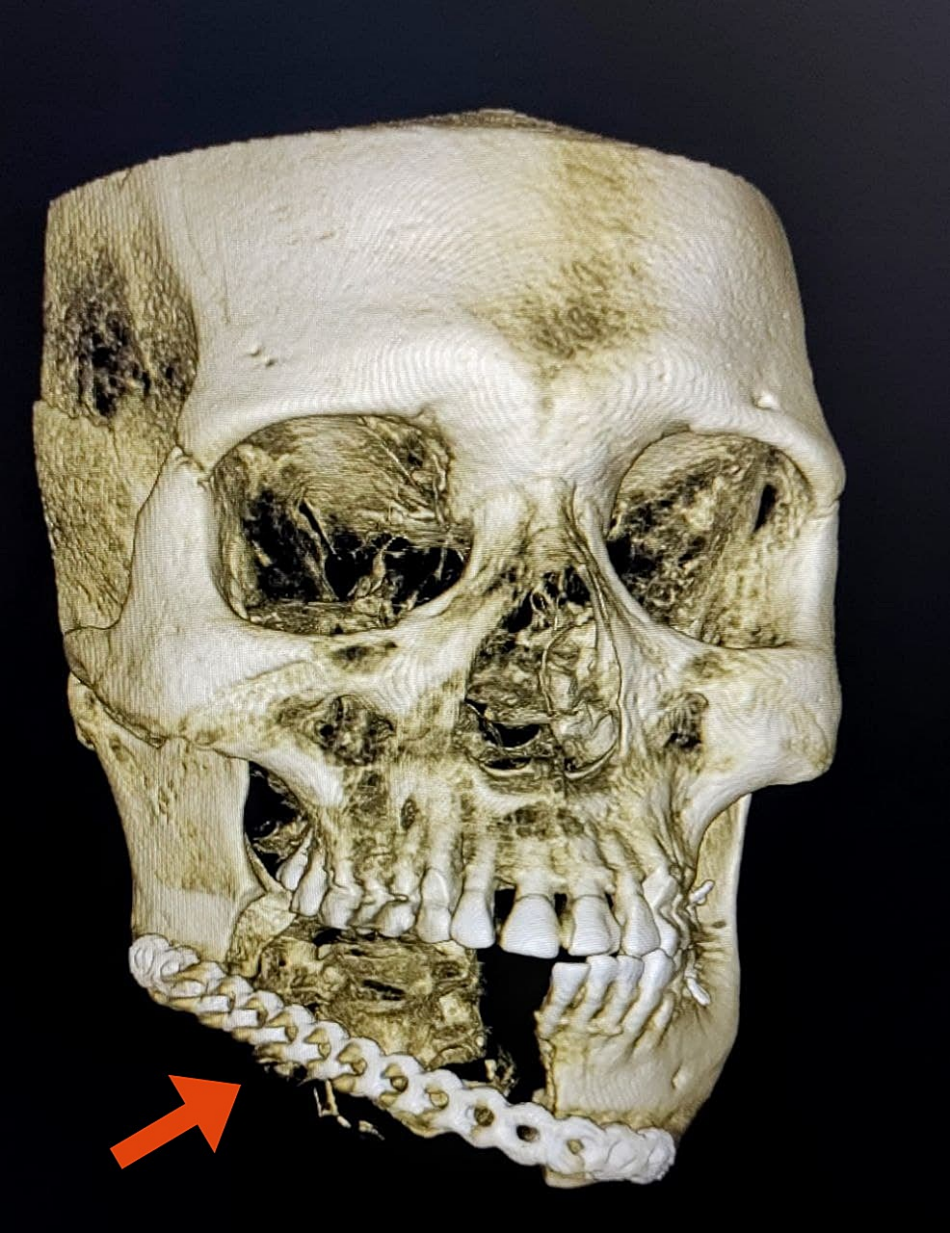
Cone-beam computerized tomography scan revealing stability of the recon plate over the defect at one-year postoperative follow-up period.
